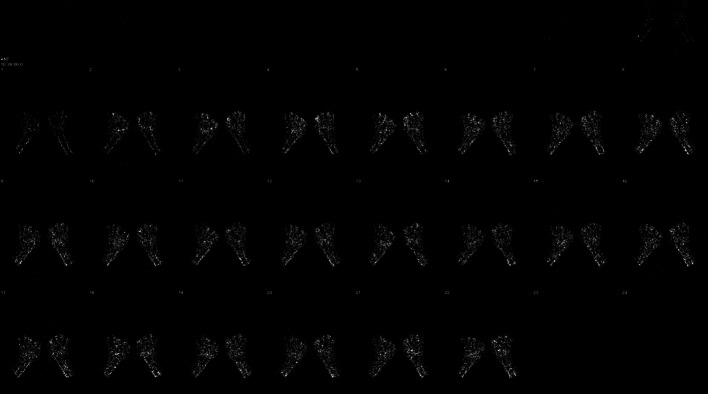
Dynamic multiphase 99mTc-MDP bone scintigraphic. Images of the hands show absent tracer uptake throughout the bilateral phalanges in the palmar blood flow phase immediately after the radiotracer injection
